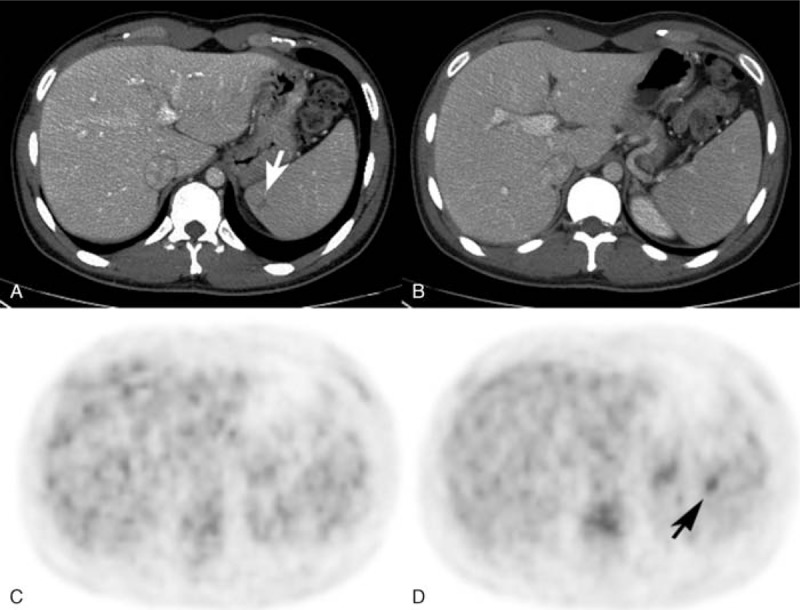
(A) A patient with a hypodense HD lesion in the spleen (arrow) and (B) another patient with no abnormalities in ceCT. (C) No pathological ^18^F FDG uptake was detectable in the patient shown in (A) and (C), while in (D) a focal lesion was detectable in PET/ldCT (arrow) in the patient with a normal ceCT scan in the spleen (B). All these findings were not detectable after 2×ABVD cycles (see text).
